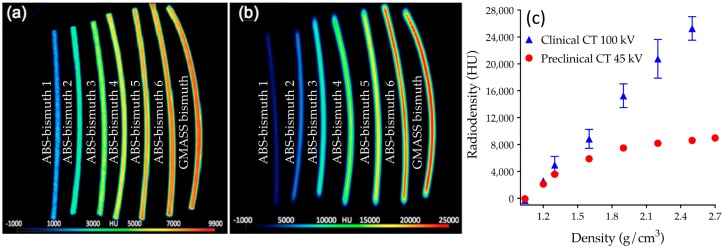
Radiodensities of 3D-printed filaments: Reconstructed image slice of preclinical CT (a) and clinical CT (b) data of ABS-bismuth and GMASS filaments with colorimetric representation of radiodensity; (c) Volume of interest data of radiodensities were plotted as a function of filament density for ABS, ABS-bismuth, and GMASS filaments from both preclinical and clinical CT scans.
